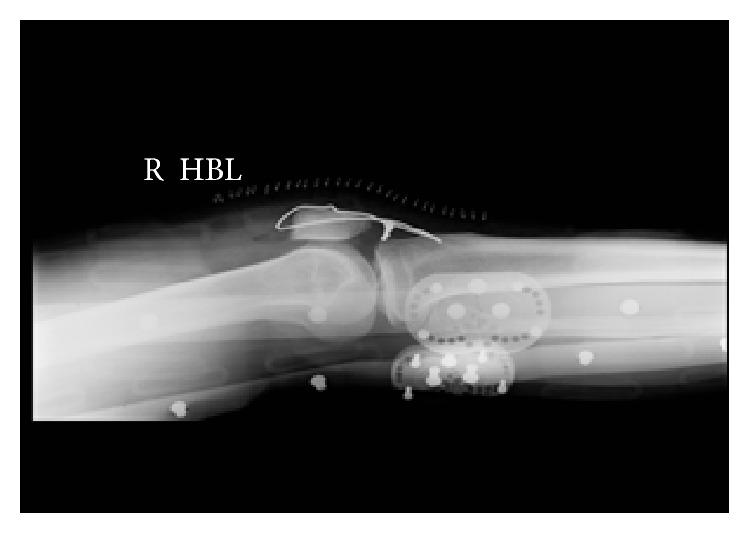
Postoperative right knee radiograph.
